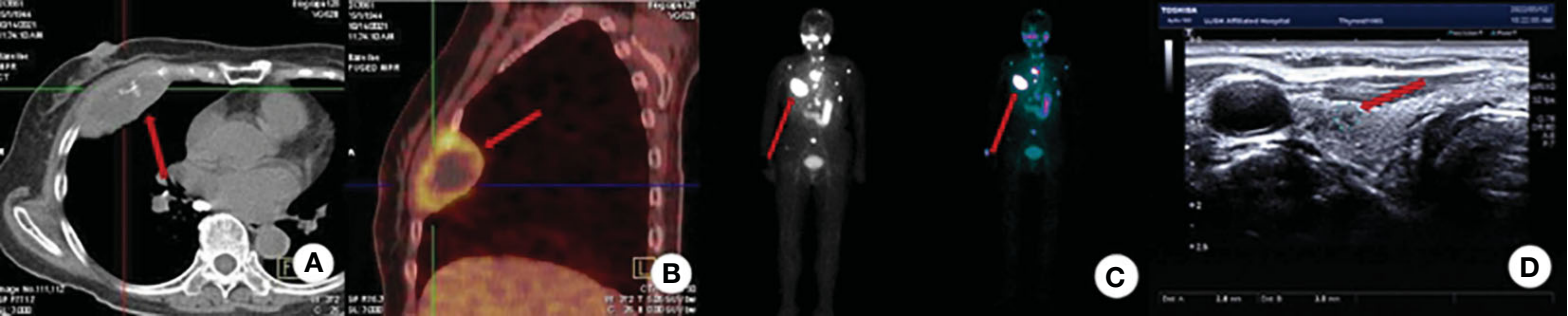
Imaging examination. (A) CT (axial view): Bone destruction of the 4th rib on the right side with a soft tissue mass shadow; (B) PET-CT: bone destruction of the right 4th anterior rib and T5 left arch with soft tissue mass shadow with increased metabolic activity, consider MT; (C) SPECT: Detection of multiple nucleoid-concentrated shadows in the field of view is considered for uptake of TC bone metastases. (D) Thyroid ultrasound: A nodule in the right lobe of the thyroid: C-TIRADS: Class 4A.
